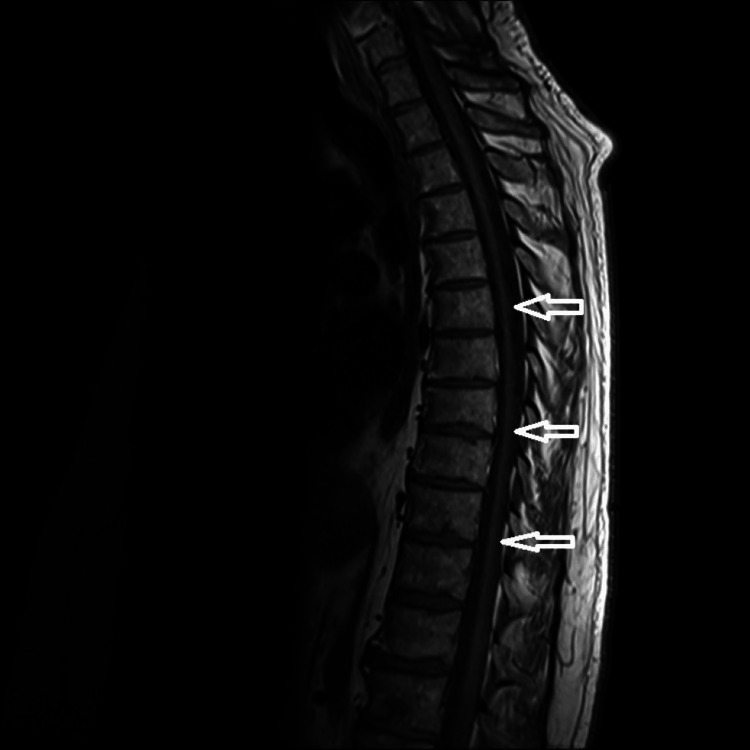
MRI thoracic spine, sagittal view, T1-weighted image, showing normal spinal cord (white arrows) without cord compression or signal changes. MRI - magnetic resonance imaging
